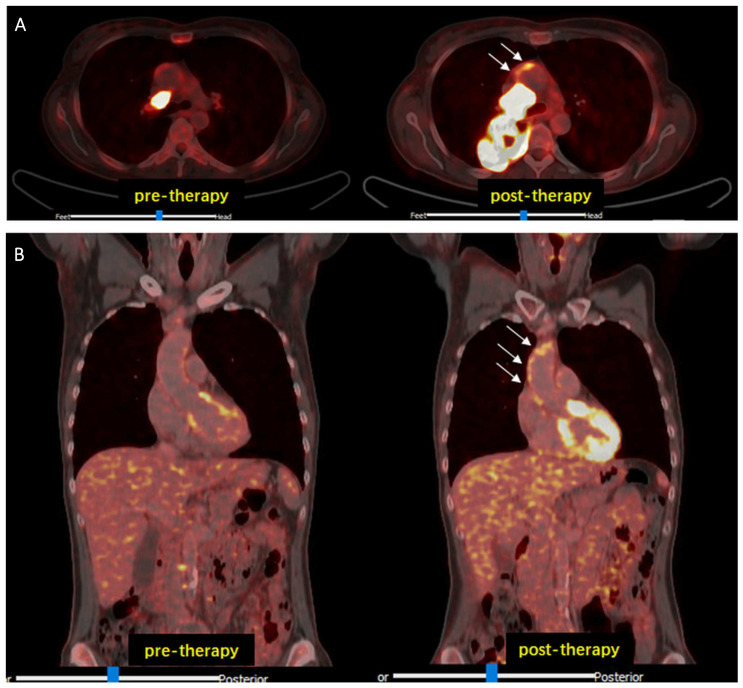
Representative 2-[18F]FDG PET/CT images. Increased arterial 2-[18F]FDG uptake (white arrows) after ICI therapy compared to baseline examination (pre-therapy) observed in transaxial (A) and in coronal (B) views. Abbreviations: CT, computed tomography; 2-[^18^F]FDG, 2-[^18^F]fluorodeoxyglucose; ICI, immune checkpoint inhibitors; PET, positron emission tomography.
